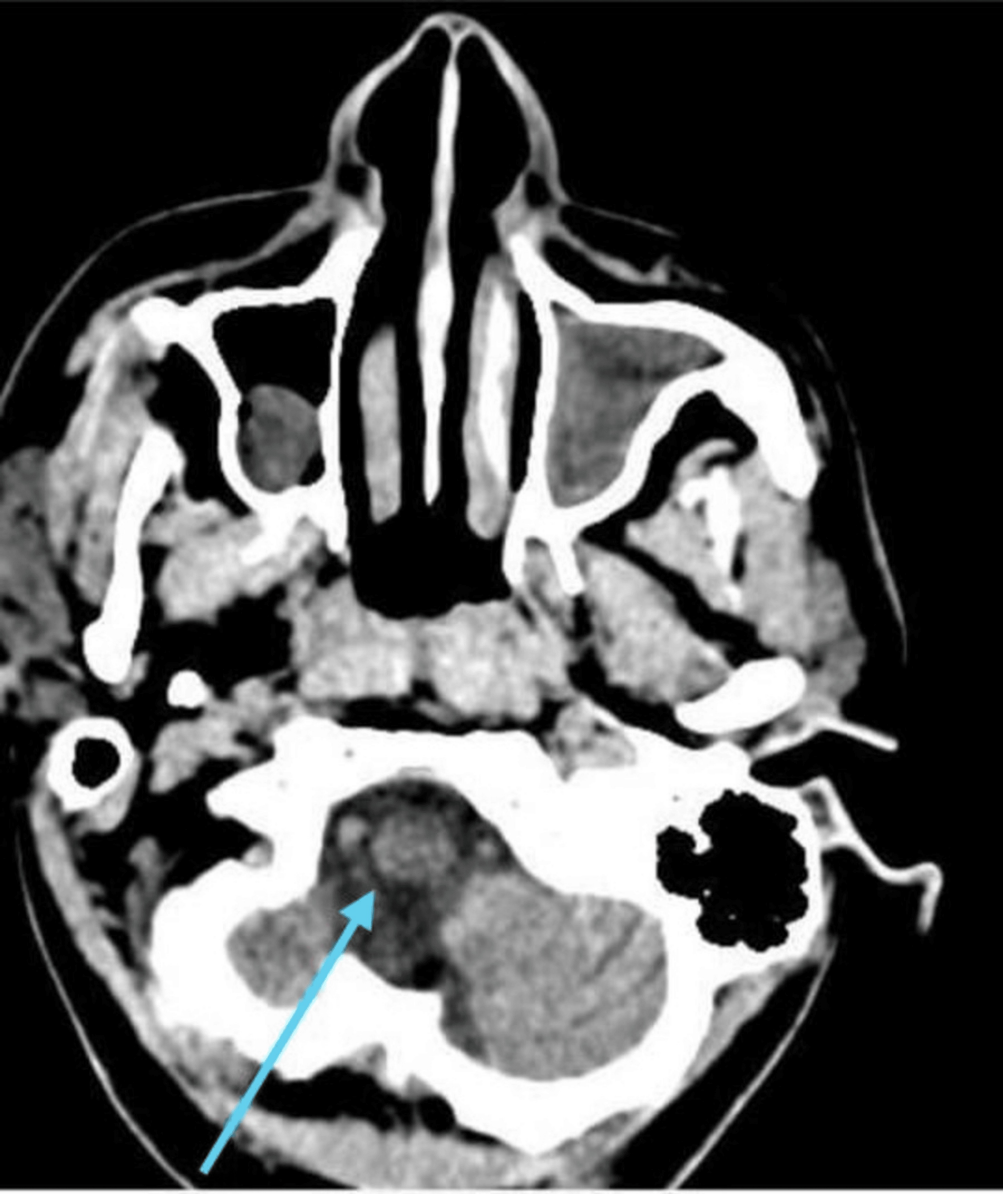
MRI of the brain The blue arrow shows a portion of the chronic infarct. MRI: Magnetic resonance imaging
